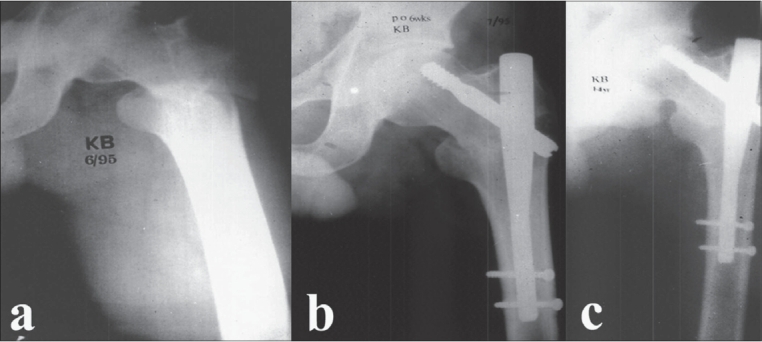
X-ray left hip antero-posterior view (a) Trochanteric fractures (A3/AO classification) left femur showing reverse obliquity. (b) Postoperative radiograph showing fracture well fixed with gamma nail showing good alignment and position. (c) 2 year follow up radiograph showing consolidation at fracture site
